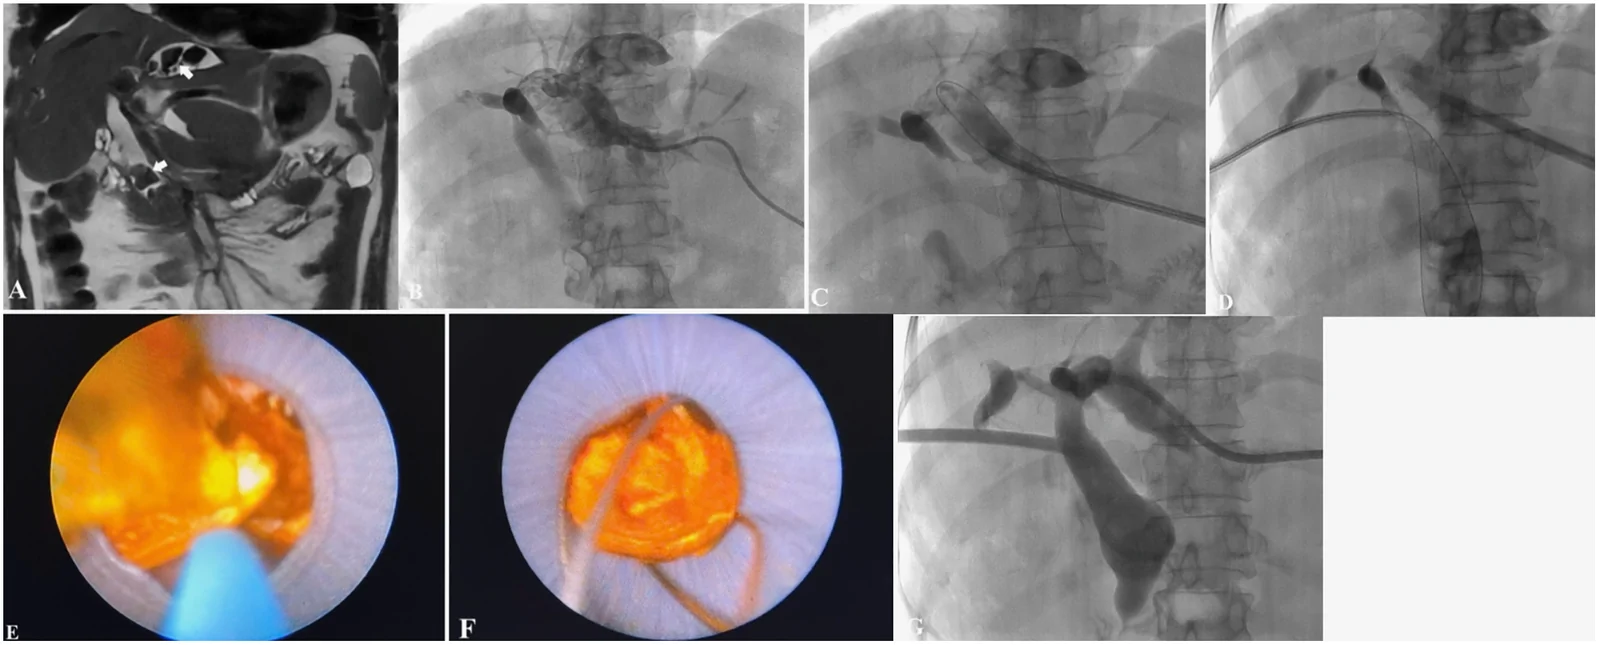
An 87-year-old woman presented with a 3-day history of abdominal pain, fever, and jaundice, diagnosed as Grade III acute cholangitis per Tokyo Guidelines. (A) Coronal T2-weighted MRI revealed multiple stones in the left hepatic duct and common bile duct (arrowhead). (B) PTBD was performed for decompression and bile culture. (C) After 7 days, percutaneous transhepatic endoscopic holmium laser lithotripsy (PTEHL) was initiated; the tract was dilated to 16F and an Amplatz sheath inserted. (D) A second tract was established into the common hepatic duct. (E-F) Holmium laser fragmented large stones, which were extracted with a retrieval basket. (G) Final cholangiography showed complete stone clearance.
